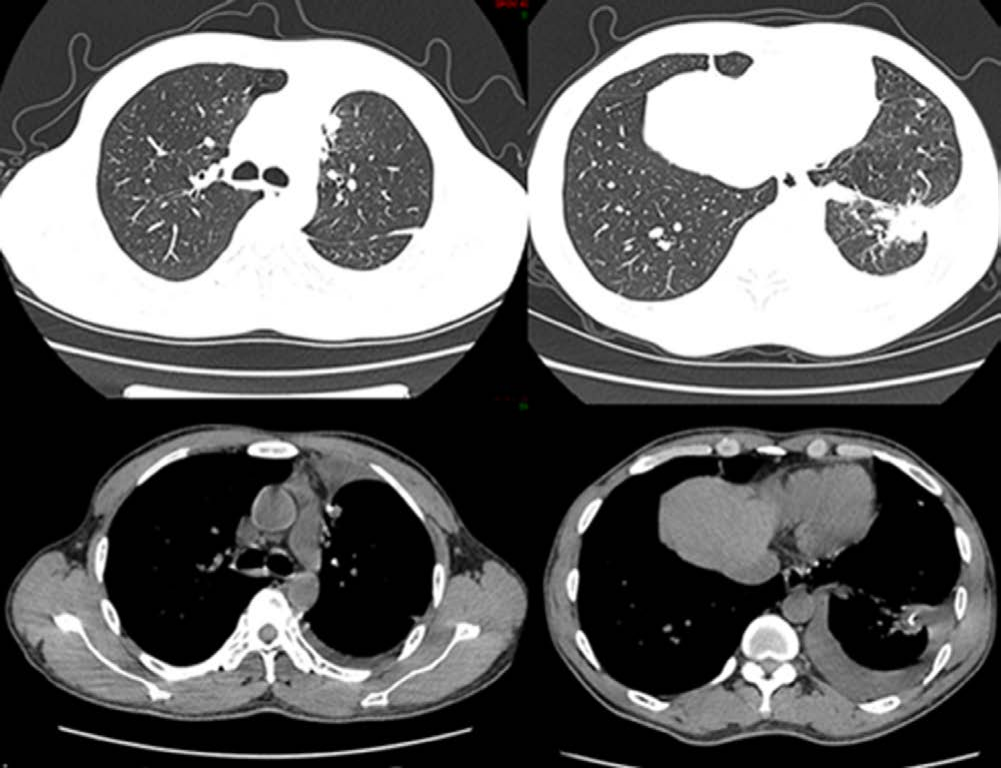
Postoperative follow-up CT scan (January 23, 2025). CT = computed tomography.
